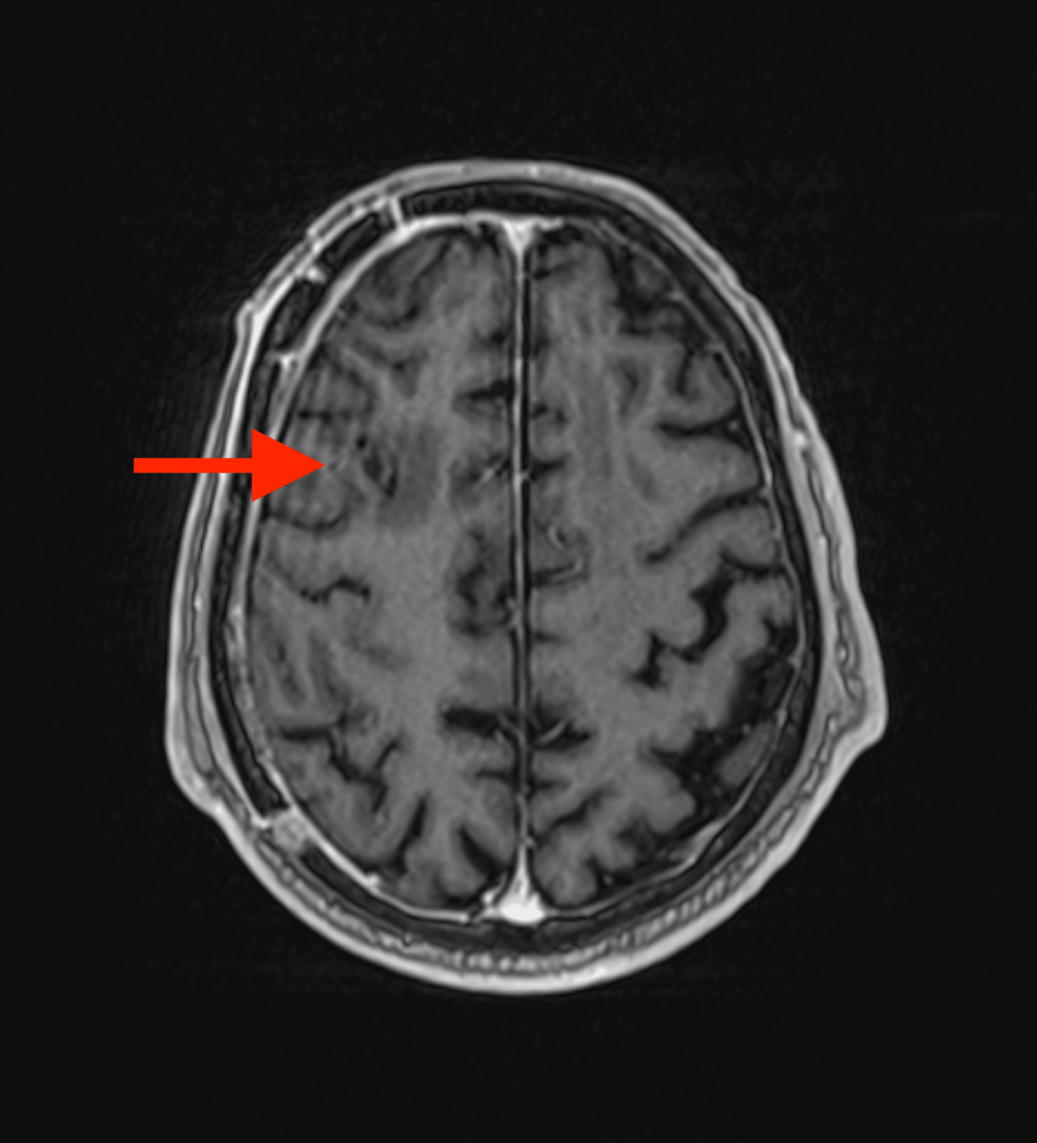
MRI of the brain showing resolved right frontal abscess, 10 weeks after surgery
